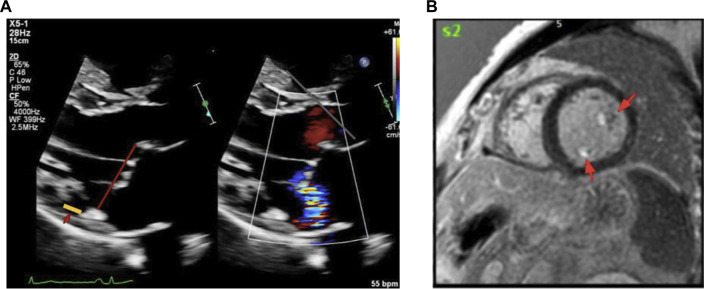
Transthoracic echocardiography (TTE) and Cardiac MRI Showing Fibrosis in Mitral Valve Prolapse. (A) shows the parasternal long-axis view during end-systole shows bileaflet mitral valve prolapse with MAD, highlighted by the yellow line. (B) shows cardiac MRI demonstrates late gadolinium enhancement in the papillary muscles and mitral annulus, indicating areas of fibrosis in a patient with malignant mitral valve prolapse. Abbreviations: MAD, mitral annular disjunction; MRI, magnetic resonance imaging.
